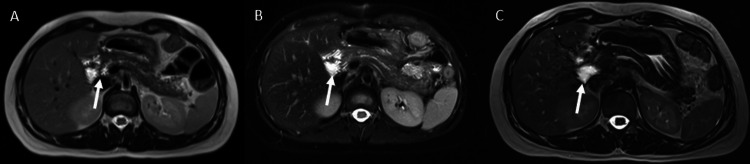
Postoperative magnetic resonance imaging demonstrating changes after pancreatoduodenectomy (A–C) Axial magnetic resonance images obtained during postoperative follow-up showing expected postsurgical changes in the pancreatic head region following pancreatoduodenectomy. Areas of altered signal intensity (arrows) correspond to postoperative changes, including fibrosis and surgical reconstruction. No discrete mass lesion is identified, and there is no radiological evidence of tumor recurrence or metastatic disease.
